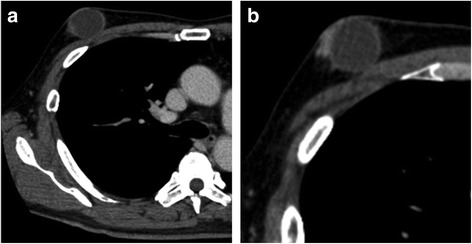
CT image findings: Contrast-enhanced computed tomography confirmed thickening of the wall that protruded outside the cyst (a, b)
